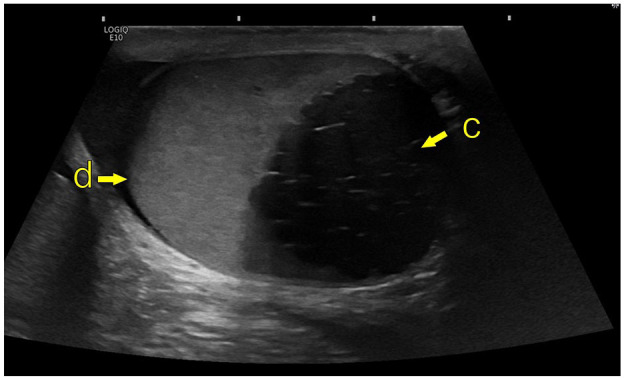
Ultrasound male genital organs; GE Logique10 (GE HealthCare); linear transducer ML6-15. (d) Left testicle, (c) Cystic enlargement of the left seminal vesicle.
